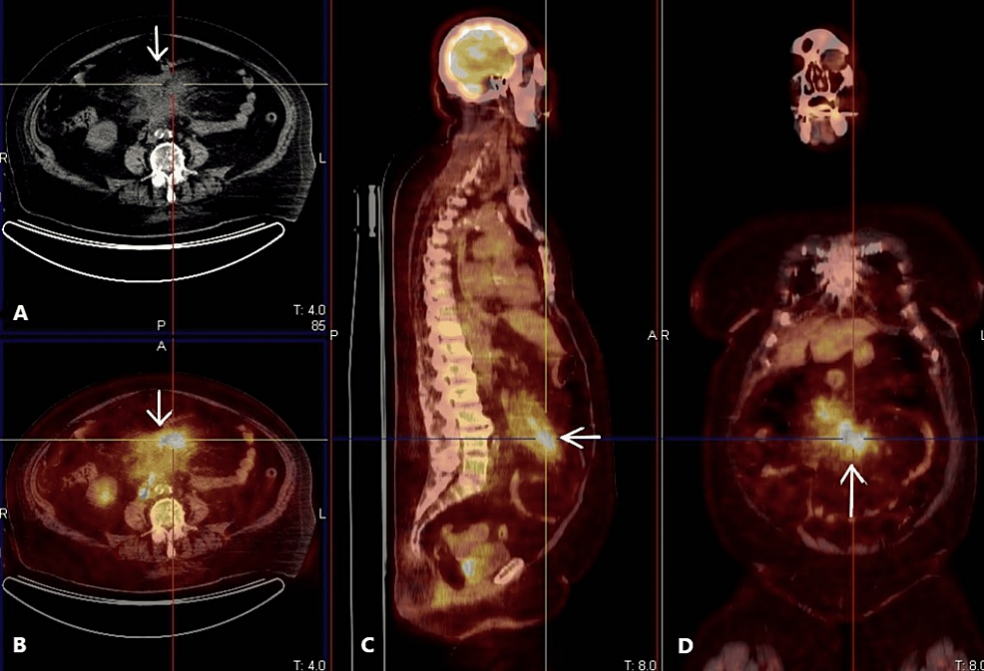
Pre-operatory imaging study of our patient with CMCT and total body 18F-FDG PET/CT. (A) Axial view of CMCT, contrast medium computed tomography, showing a swelling of the mesenteric root with slightly contrast-impregnated tissue and blurred and irregular margins (see arrow). (B) Axial view, (C) sagittal view, and (D) coronal view of total body 18F-FDG PET/CT, 18F-fluoro deoxy glucose positron tomography/computed tomography, showing an area of inhomogeneous and intense hypermetabolism of the mesentery root (see arrows).
